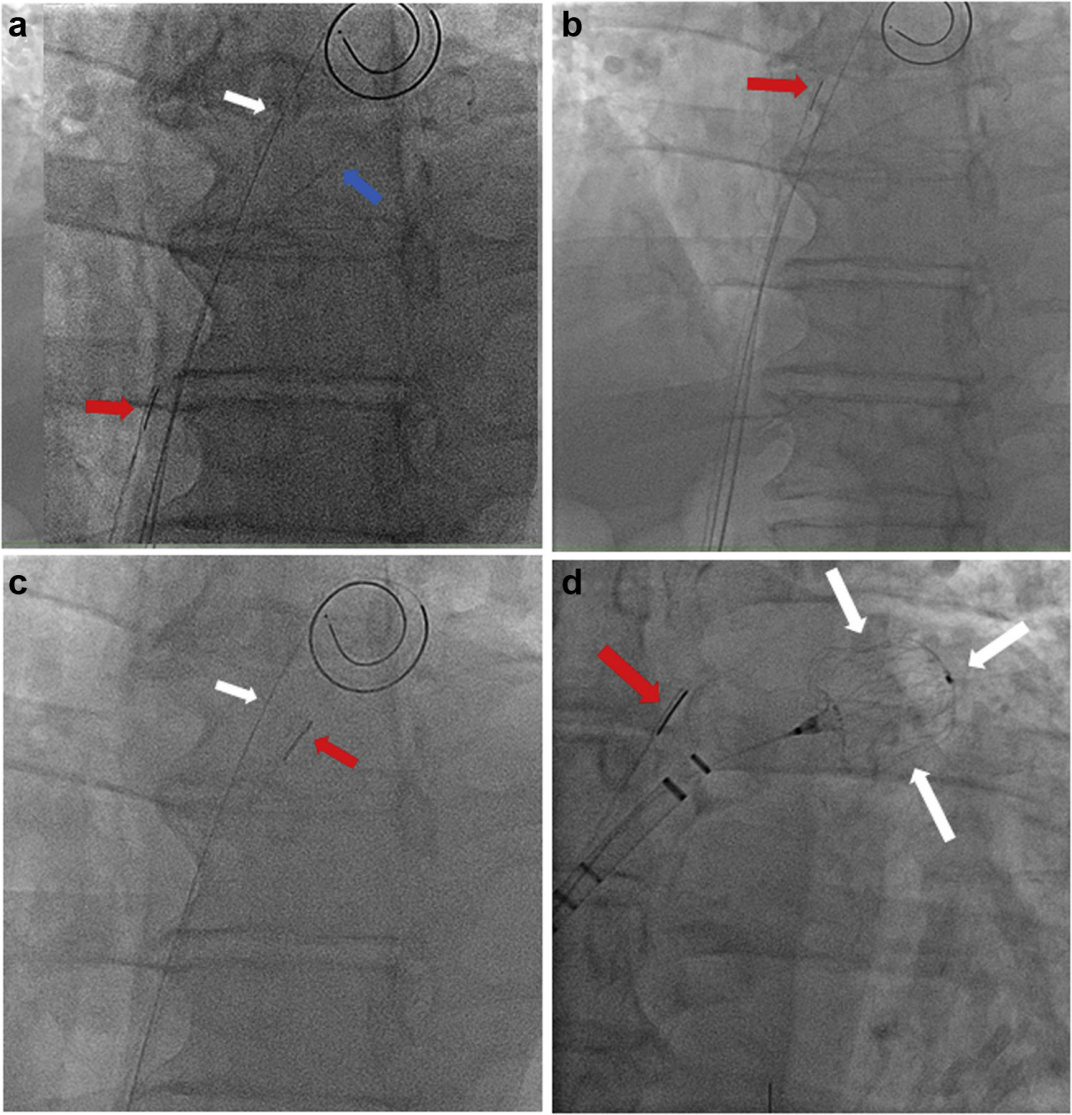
Angiography showing the dual guidewire technique to cross the intracardiac echocardiogram (ICE) probe through the transeptal puncture for left atrium appendage closure (LAAC). (a) ICE probe (red arrow), Bailys wire (white arrow) and Amplatz guidewire (blue arrow). (b) ICE probe (red arrow) freely inside the left atrium. (c) Bailys wire (white arrow) and ICE probe (red arrow) freely inside the left atrium after removal of the Amplatz guidewire. (d) Angiography showing successful final result and confirming the good position and compression after deployment of the WATCHMAN device. White arrows: LAA WATCHMAN closure device; Red arrow: ICE probe.
